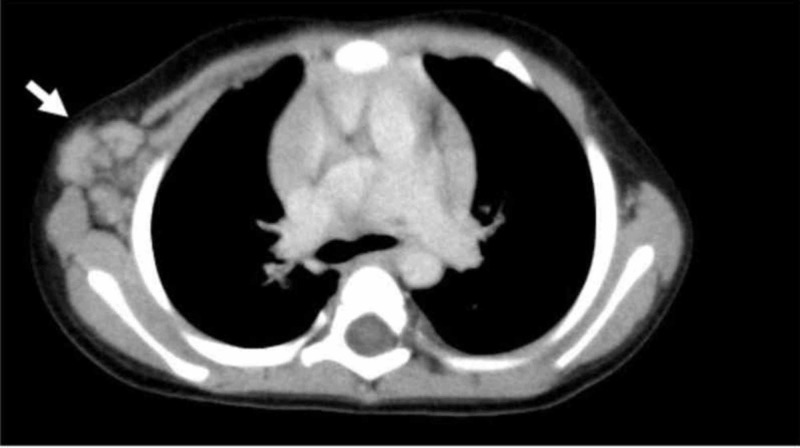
CT Scan of the Chest Wall Axial image from a scan with mediastinal windows discloses swelling of the right lymph nodes and their spreading along the chest wall (arrow).
